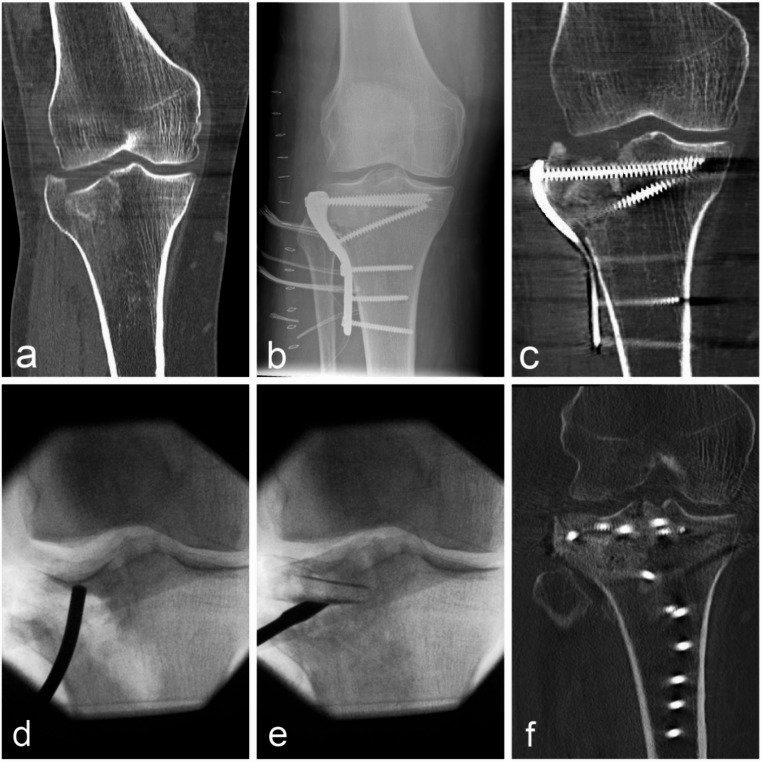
(a) Pre-operative CT scan of a 57-year-old male patient who was kicked by a bull and sustained a proximal tibia fracture type II according to the Schatzker classification. (b) The initial fracture was treated with open reduction and internal fixation using a lateral locking plate at another institution. However, the patient subsequently presented to our center with persistent stabbing pain and discomfort that had not improved despite pain management and physical therapy. (c) CT scans performed at our center revealed a depression of the lateral tibial plateau by 5.3 mm, primarily in the dorsal region. (d) Four weeks later, revision surgery was undertaken. The initial fixation material was removed, and an arthroscopically assisted reconstruction of the articular surface was performed. The entire dorsolateral quadrant was elevated by 5 mm. (e) The defect was then filled with both an allogenic tricortical bone chip and allogenic spongiosa. (f) Postoperatively, the tibial plateau was restored to its physiological height, resulting in a smooth reconstruction of the articular surface. The patient experienced complete relief from the stabbing pain and was pain-free following the procedure
